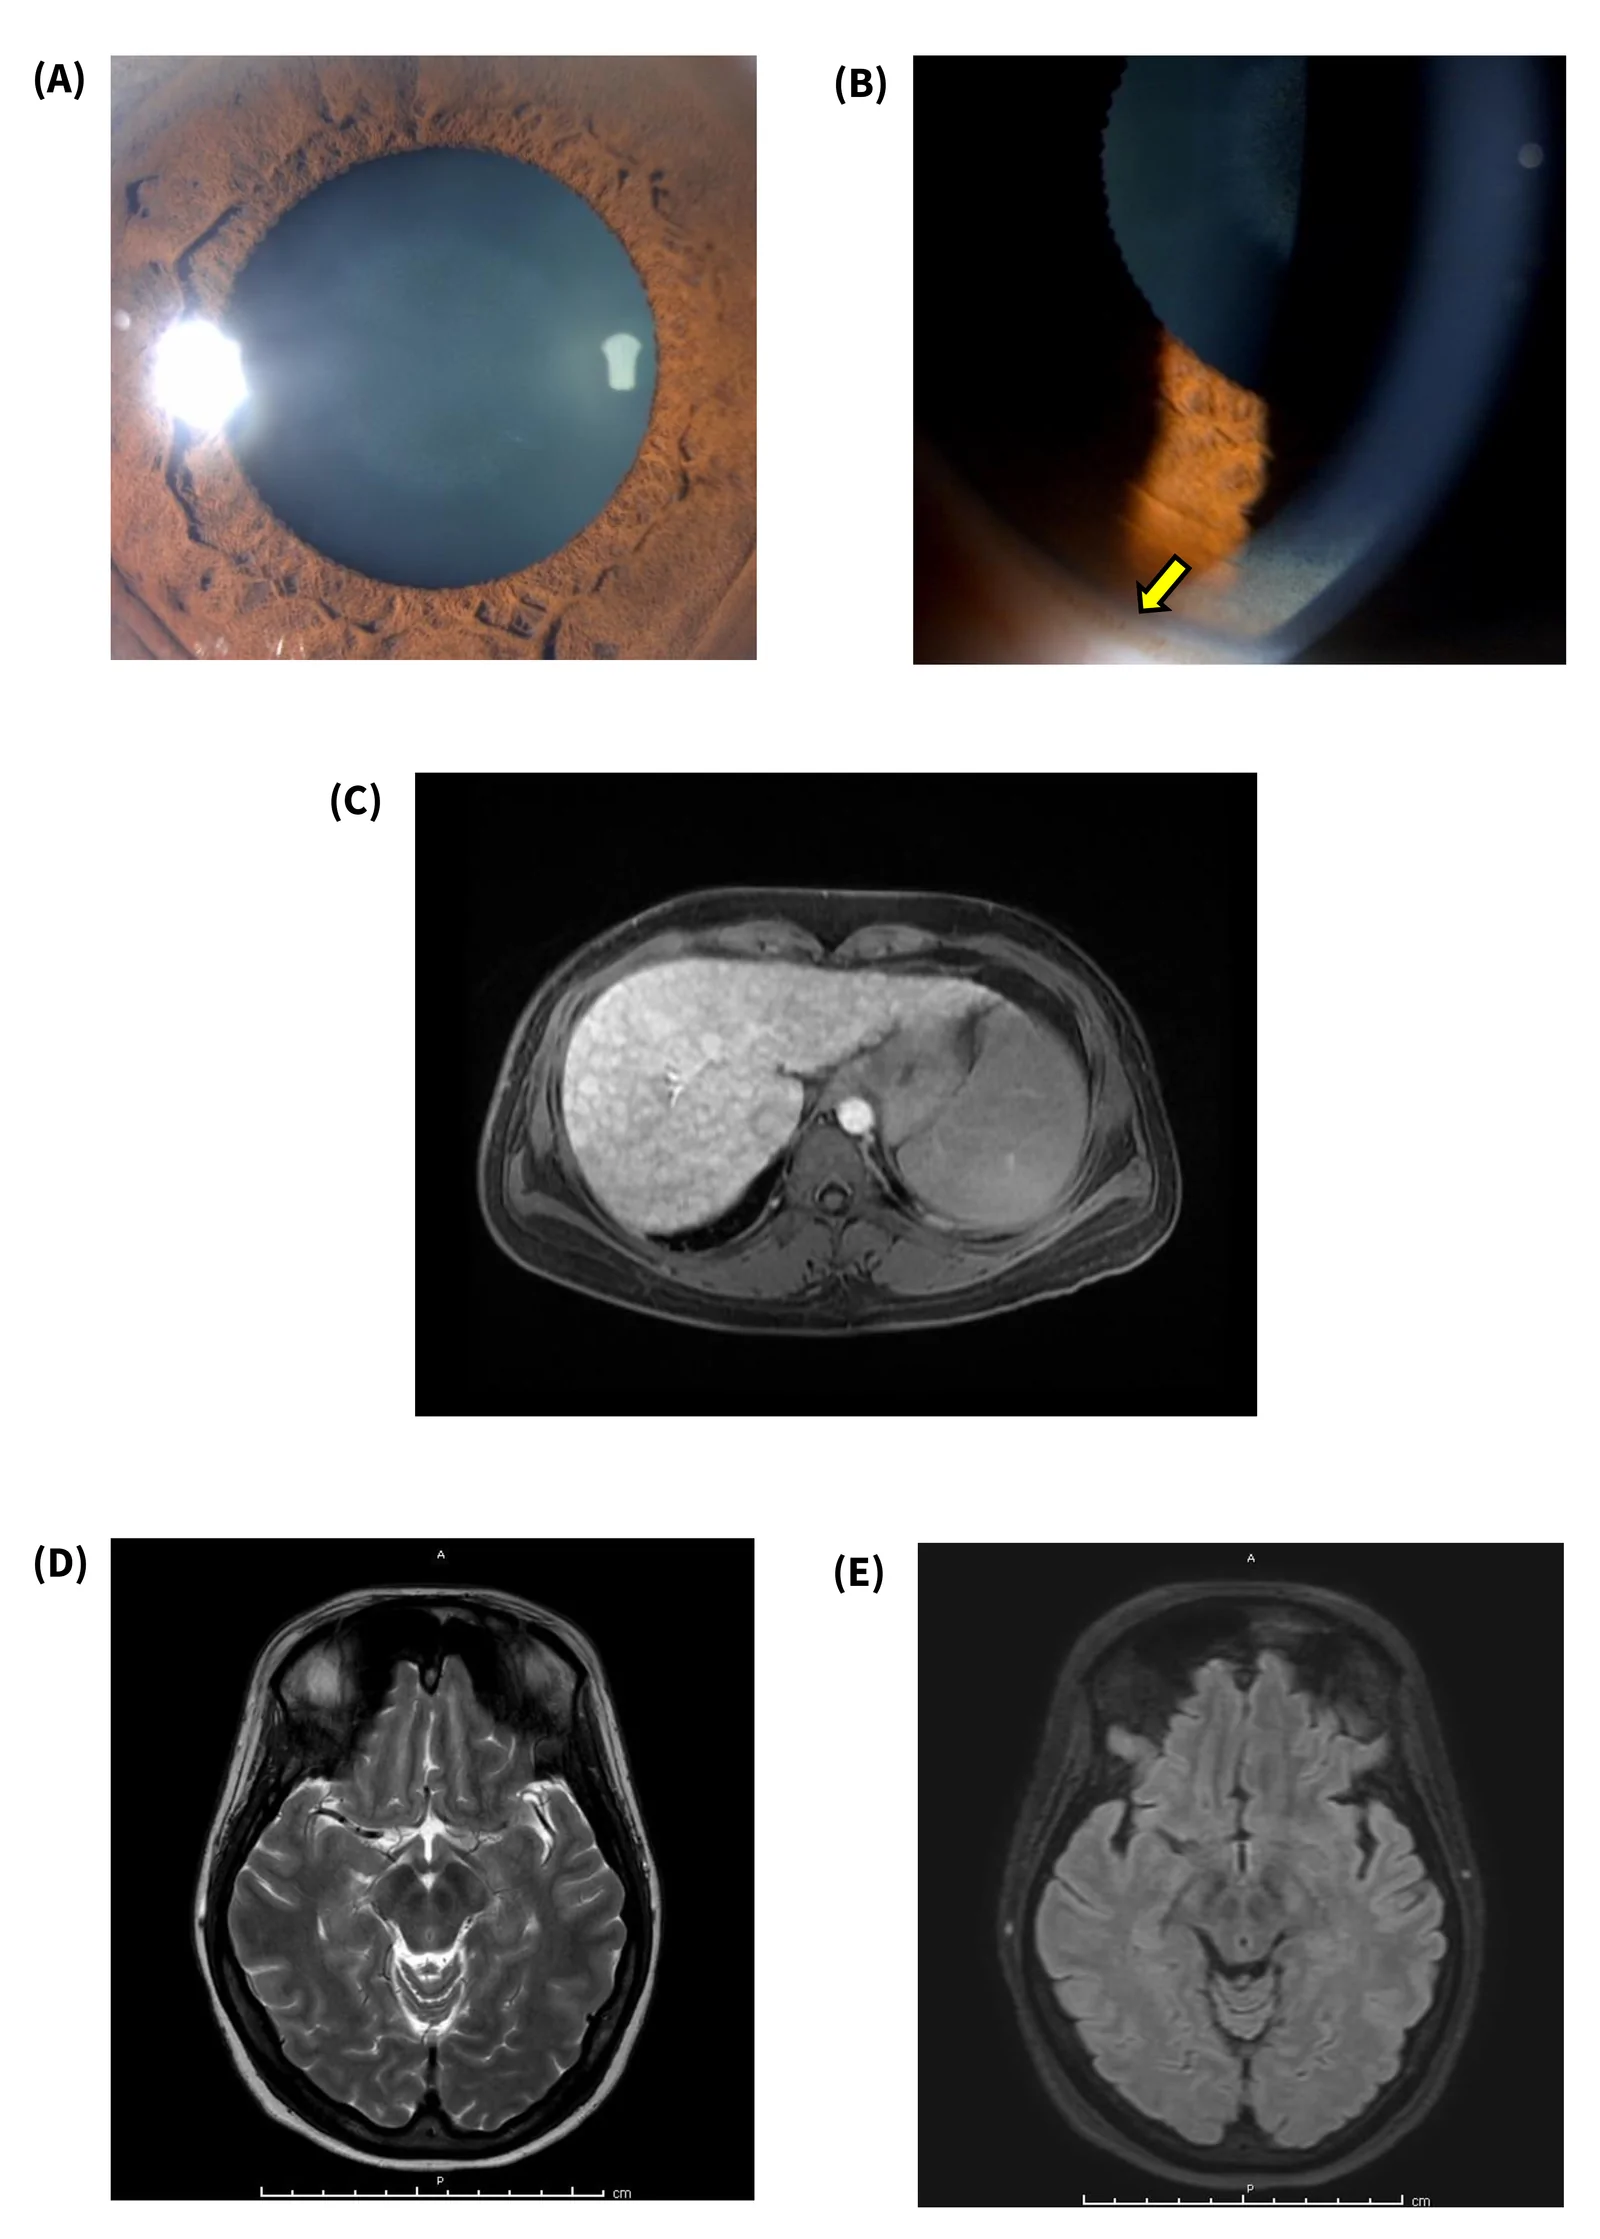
Significant clinical features of Wilson disease identified in the proband. (A) Sunflower cataract. (B) Copper deposition in the cornea (arrow). (C) Coarse liver parenchyma with a nodular hepatic surface, suspected cirrhosis, no arterial enhancing mass, and marked splenomegaly. (D) T2-weighted/fluid-attenuated inversion recovery image of the midbrain showing the “face of the giant panda” sign. (E) Bilateral symmetrical gradient-echo/susceptibility-weighted imaging hypointensities in the bilateral lentiform nuclei, prominent in the globus pallidi and dentate nuclei, with minimal hypointensities in the substantia nigra and red nuclei.
